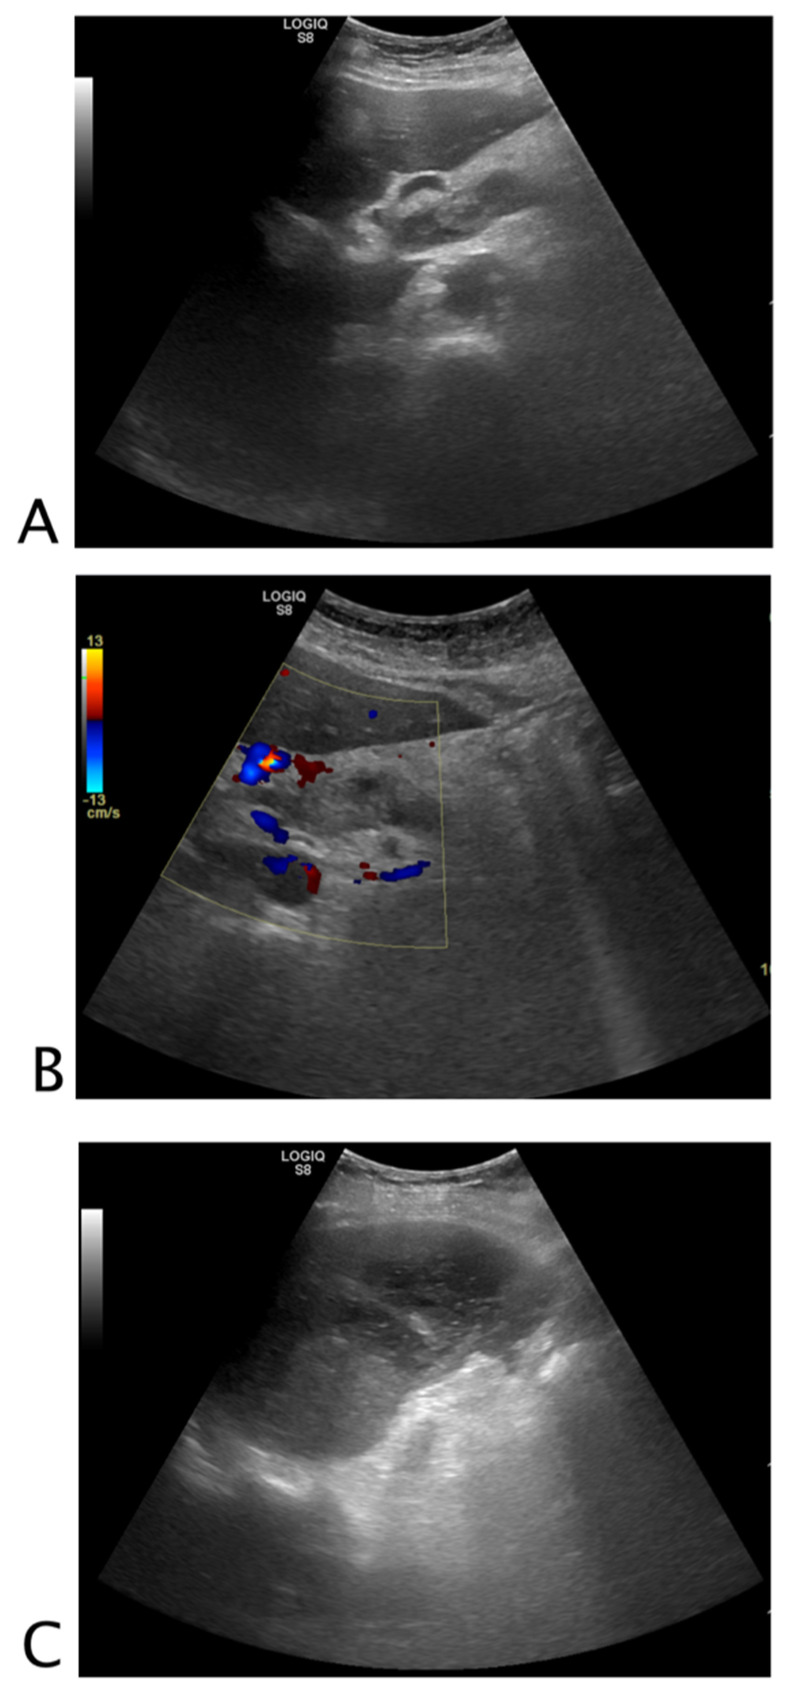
Black that is not clearly black. A 71-year-old female patient complaining of drug-resistant abdominal pain for the last three days. No history of malignancies or liver disease. At ultrasound, the portal vein was dilated and occupied by inhomogeneous echogenic material (A). The color Doppler evaluation confirmed the absence of flow in the portal vein (B). Hypoechogenic areas were detected in the spleen (C). A suspected diagnosis of portal vein thrombosis with associated spleen infarction was formulated. Final diagnosis: portal vein thrombosis in unknown thrombophilic disorder.
